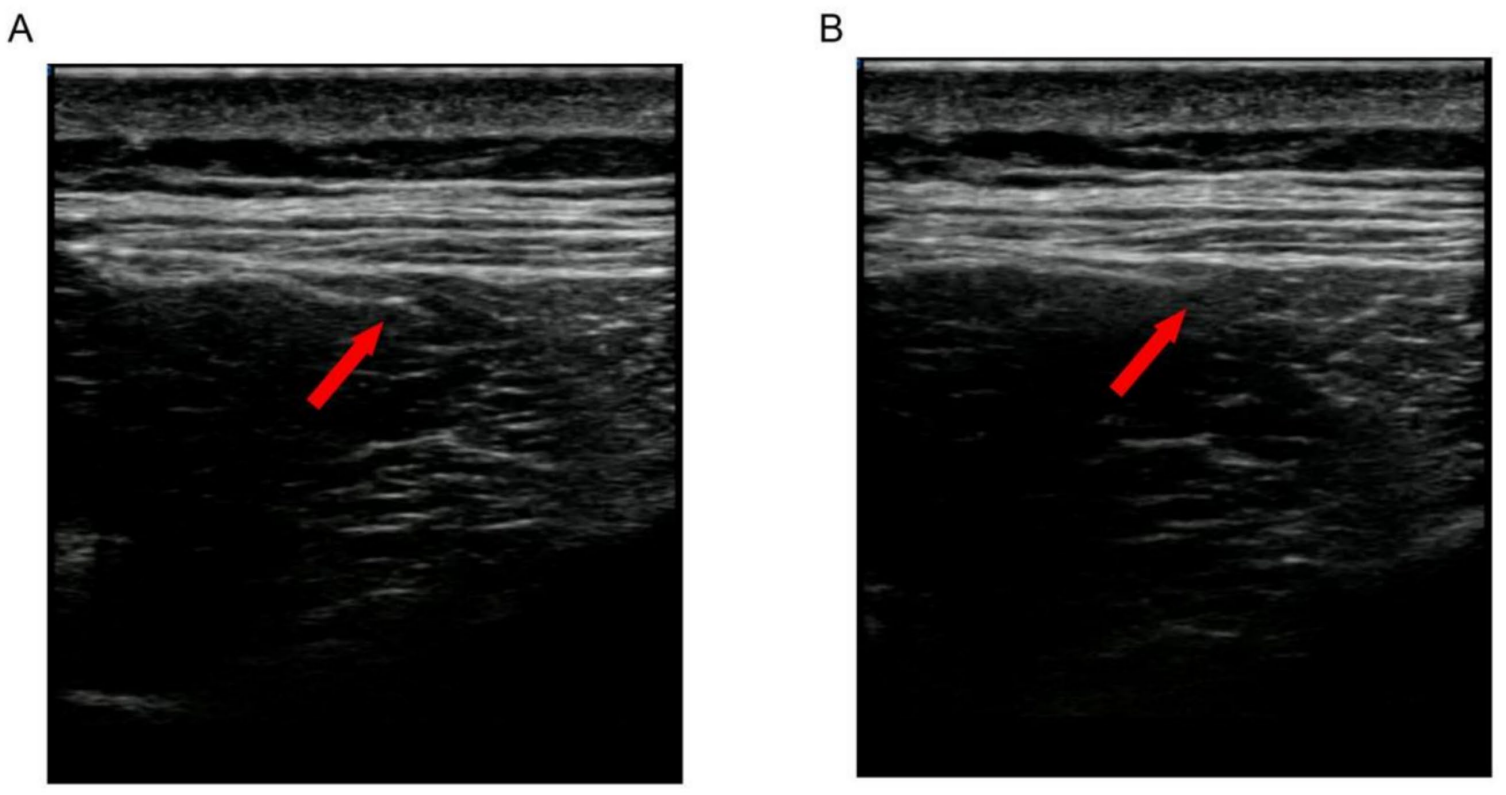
Two-dimensional ultrasonography images from the same patient before and after treatment. The red arrow: myofascial trigger points (MTrPs). (A) MTrPs showing oval, locally heterogeneous hyper echoic areas in two-dimensional ultrasound observation. (B) After repeated puncture of the MTrPs with a dry needle, the localized echoes diminished. With a dry needle, the localized echoes diminished, and the entangled muscles unraveled and relaxed.
